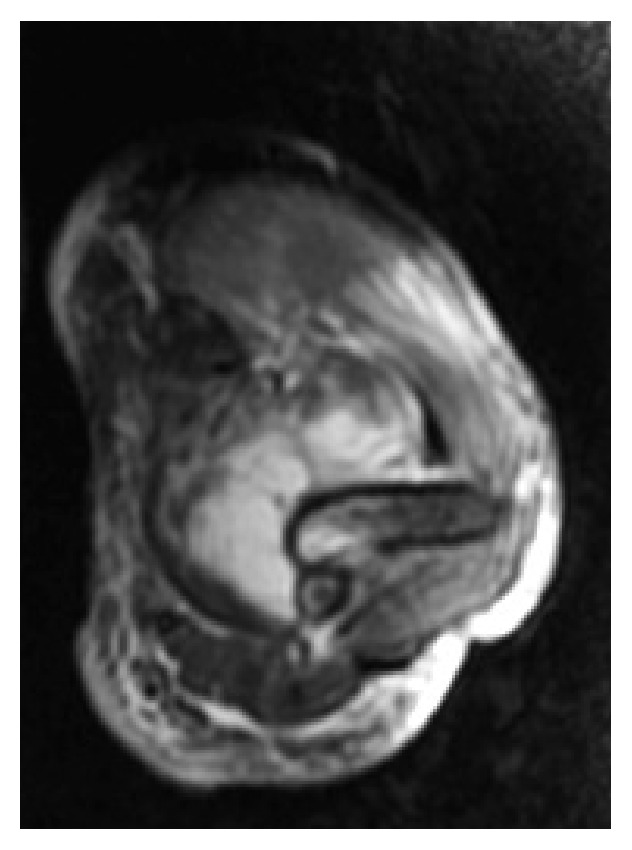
MRI STIR axial cut showing the collection.
